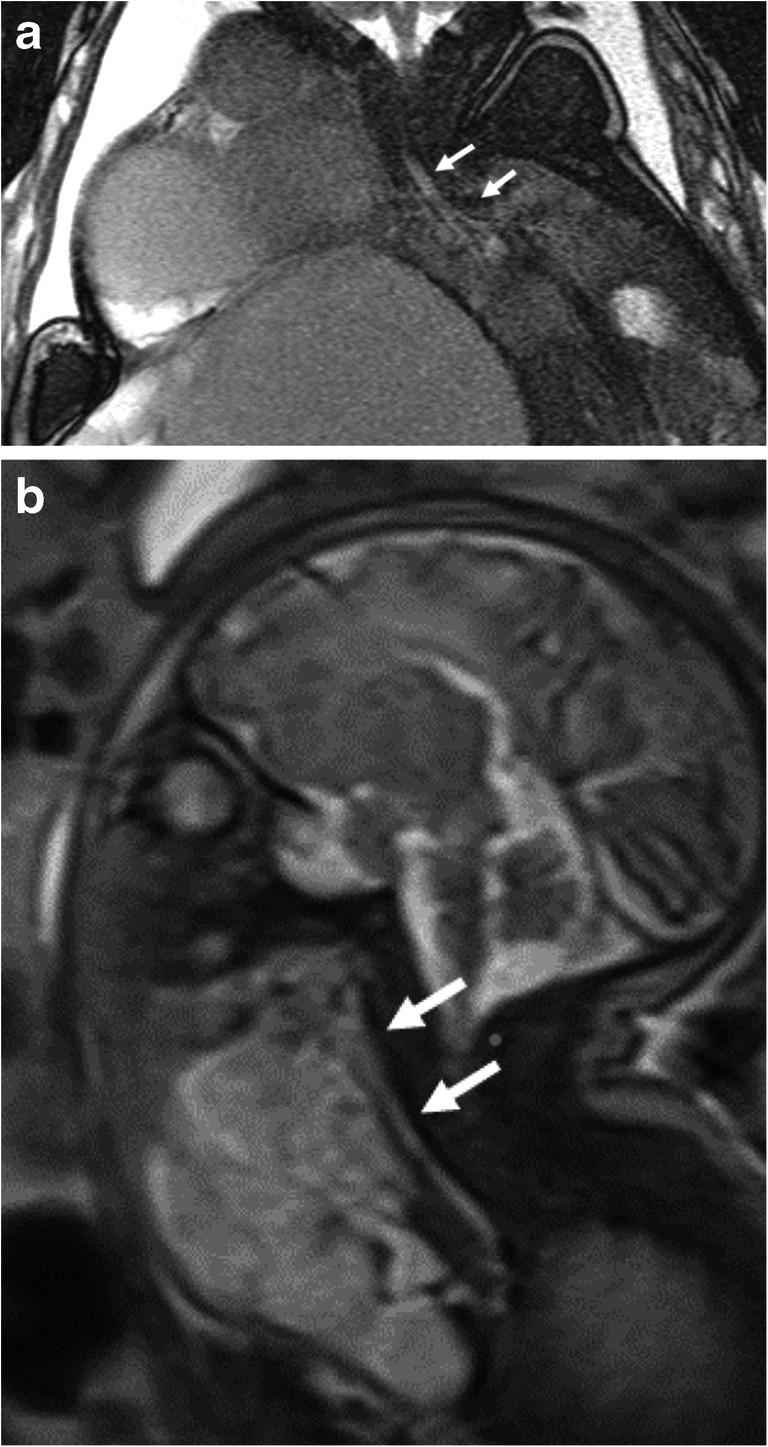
Cervical lymphatic malformations in a female fetus. a A coronal T2-weighted MR image (repetition time [TR]/echo time [TE] 6.38/3.19 ms) at 36 weeks’ gestation (N11, Tables 3 and 5) shows the voluminous macrocystic lymphatic malformation with heterogeneous signal intensity after intralesional hemorrhage. The cervical mass extends into the axilla and the thoracic and abdominal wall without invading the fetal organs. Note the normal diameter of the trachea and the main bronchi (*arrows*) and the huge abduction of the fetal arm. b In opposite, the midline sagittal T2-weighted image (TR/TE 1,200/89 ms) at 35 weeks’ gestation (N3, Tables 3 and 5) shows the anterior cervical lymphatic micro- and macrocystic malformation, extending into the submental space without invading the tongue. Note the resulting compression of the larynx and the trachea (*arrows*). An ex utero intrapartum treatment was performed at birth
